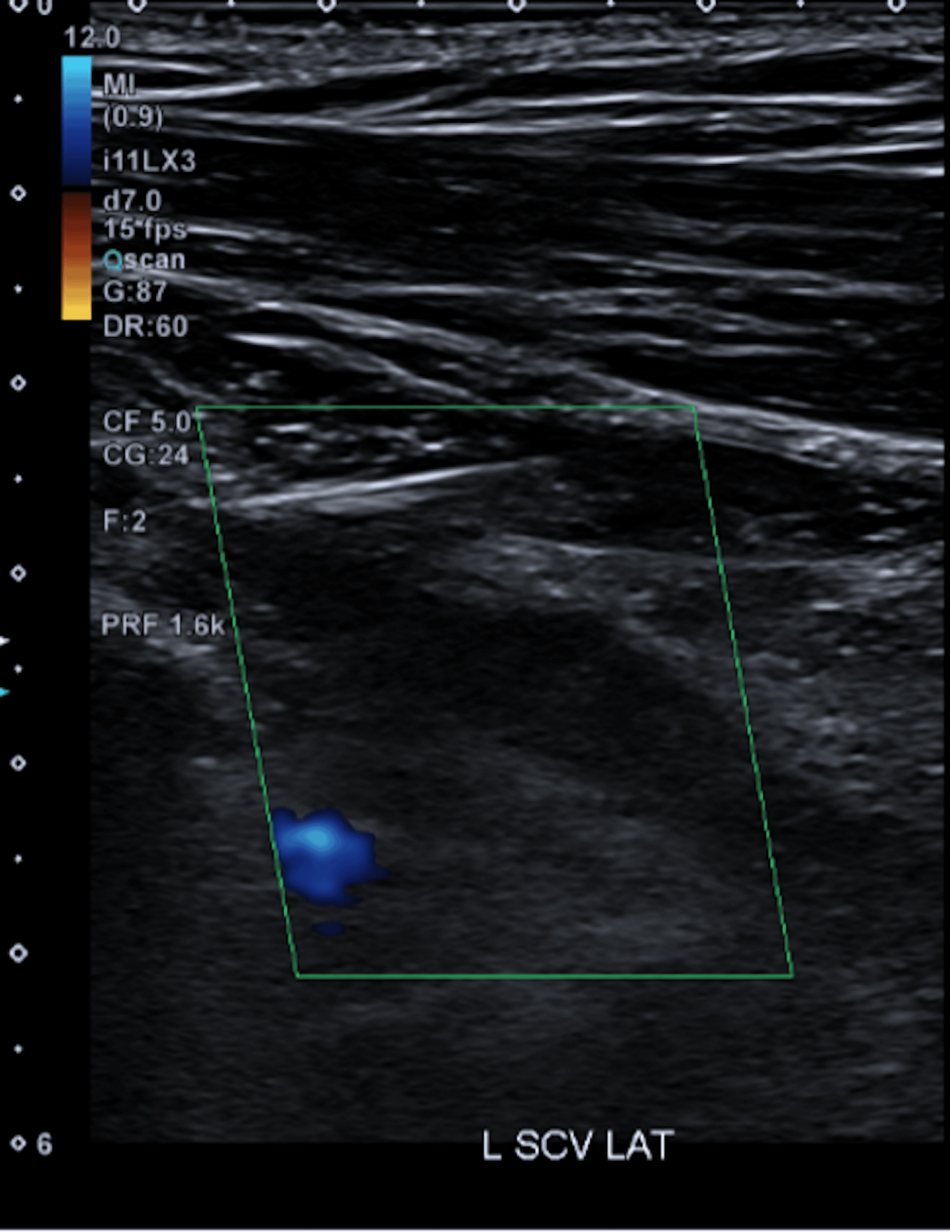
US shows no colour Doppler flow within the left subclavian vein (green box) US: ultrasound
